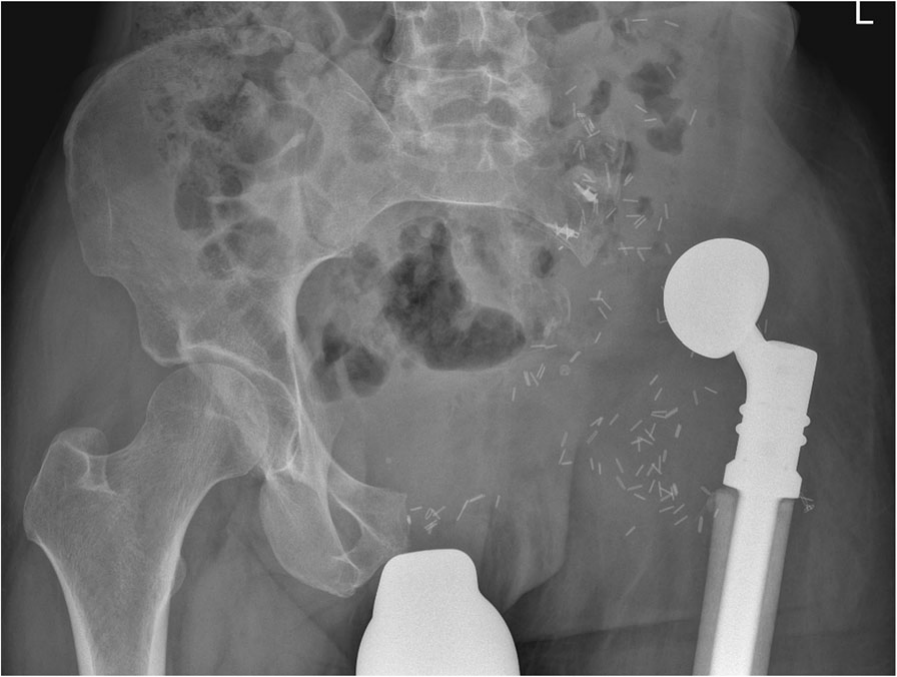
Plain radiograph of a pelvis a.p. after extraarticular, internal hemipelvectomy (types 1–3) and hip transposition. Vessel clips mark the extent of tumor resection prior to reconstruction
